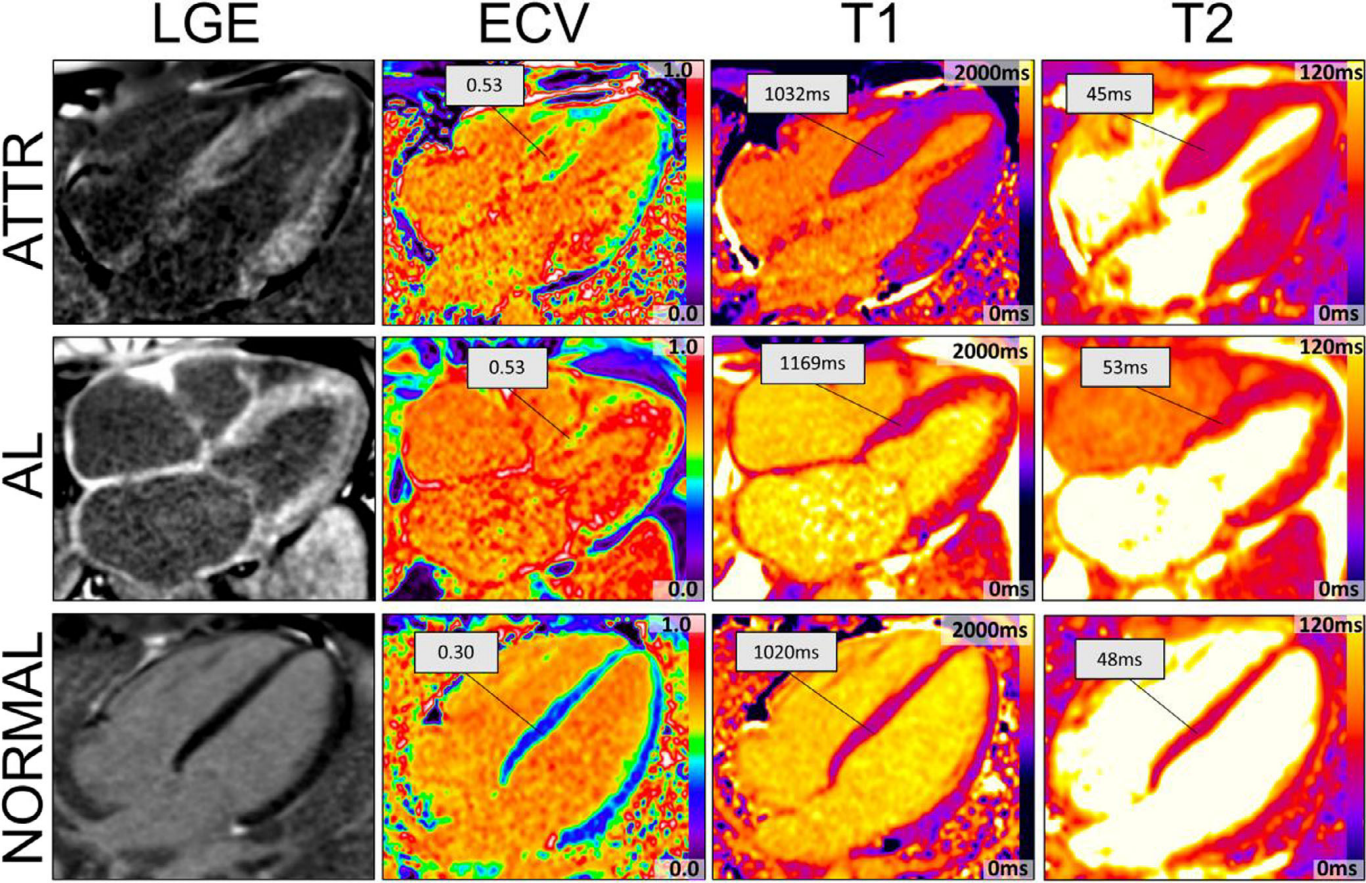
Late gadolinium enhancement (LGE), extracellular volume (ECV), native T1 and T2 maps for ATTR (top), AL (middle), and control (bottom) patients. ECV, T1, and T2 are higher in the 2 amyloid patients than in the control patient. T1 and T2 are higher in the AL than in the ATTR patient despite similar degree of cardiac amyloid infiltration (similar ECV). AL indicates light‐chain amyloidosis; ATTR, transthyretin amyloidosis; T1 and T2, magnetic fields in magnetic resonance imaging.
